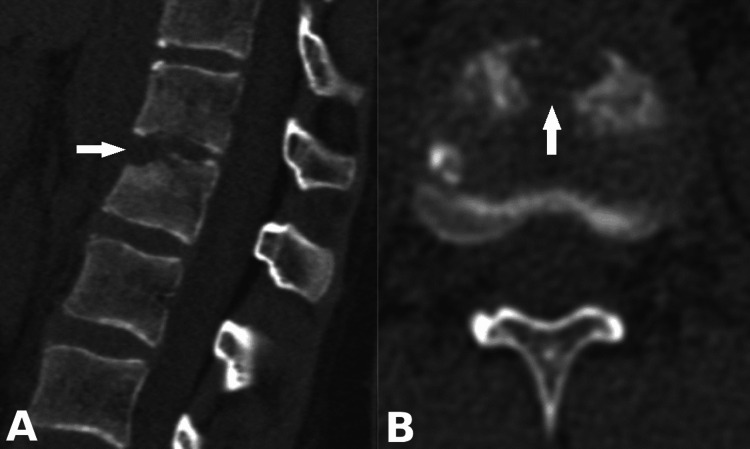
(A) Sagittal and (B) axial preoperative CT images showing the destruction of the lower endplate of T12 and upper endplate of L1 vertebral bodies (arrows)
